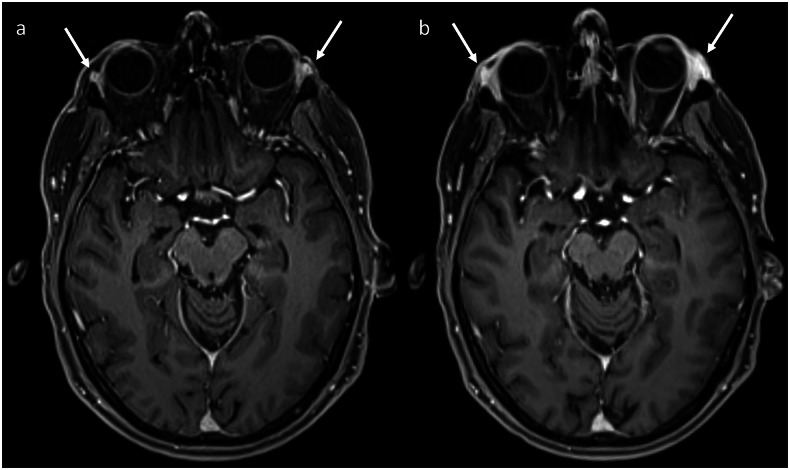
Axial post-contrast T1-weighted images before (a) and after (b) commencing ICI therapy (combination ipilimumab and nivolumab followed by maintenance nivolumab) in a patient with metastatic melanoma who developed eye pain after ICIs, demonstrating enlargement of the lacrimal glands, consistent with dacryoadenitis
